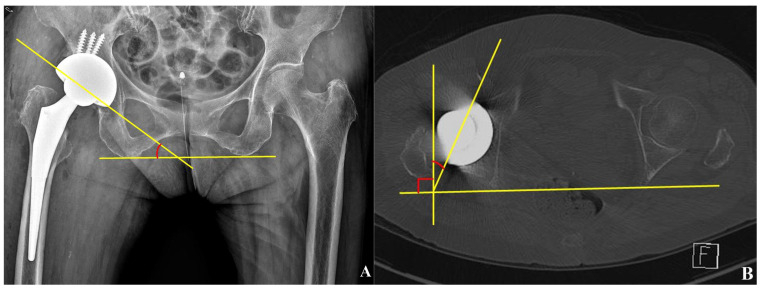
Measurement of cup inclination and anteversion. (A) Cup inclination measurement. The angle between the line tangential to the face of the acetabular cup and the line which is tangential to the inferior border of the both ischial tuberosity. (B) Cup anteversion measurement. The angle between the line tangential to the face of the acetabular cup and the line perpendicular to posterior pelvic margins at the level of the sciatic notch on axial CT scans.
